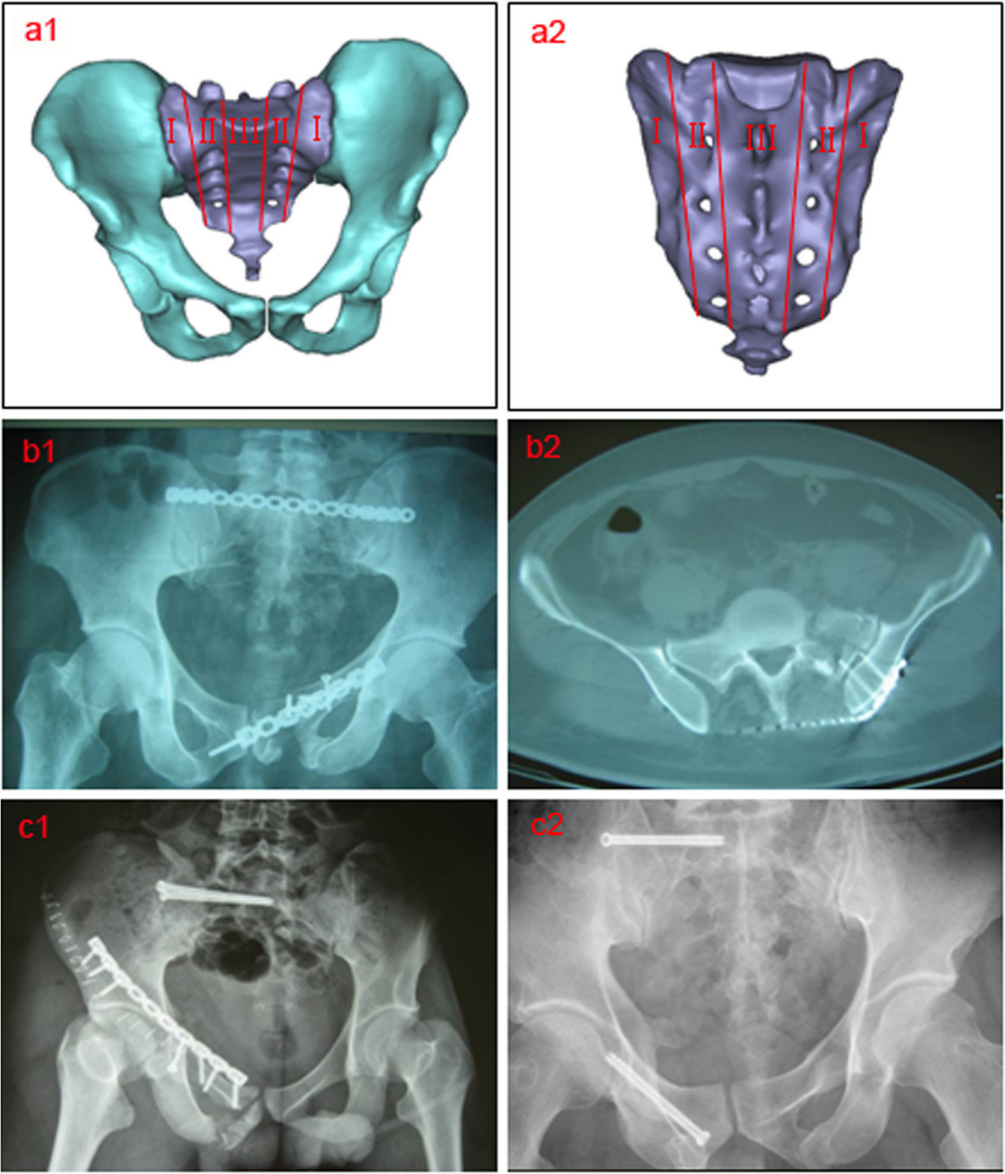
Sacral fracture typing by Denis. (a) Denis I, II and III zones of the sacrum (a1 anterior view, a2 posterior view); (b) a case (male, age 38) of a Denis II type left sacral fracture treated with percutaneous PTMP (b1 anteroposterior X-ray, b2 transverse plane CT scan); (c) a case (male, age 25) of a Denis II type right sacral fracture and a case (male, age 36) of Denis I type right sacral fracture both treated with percutaneous SIJS (anteroposterior X-ray).
